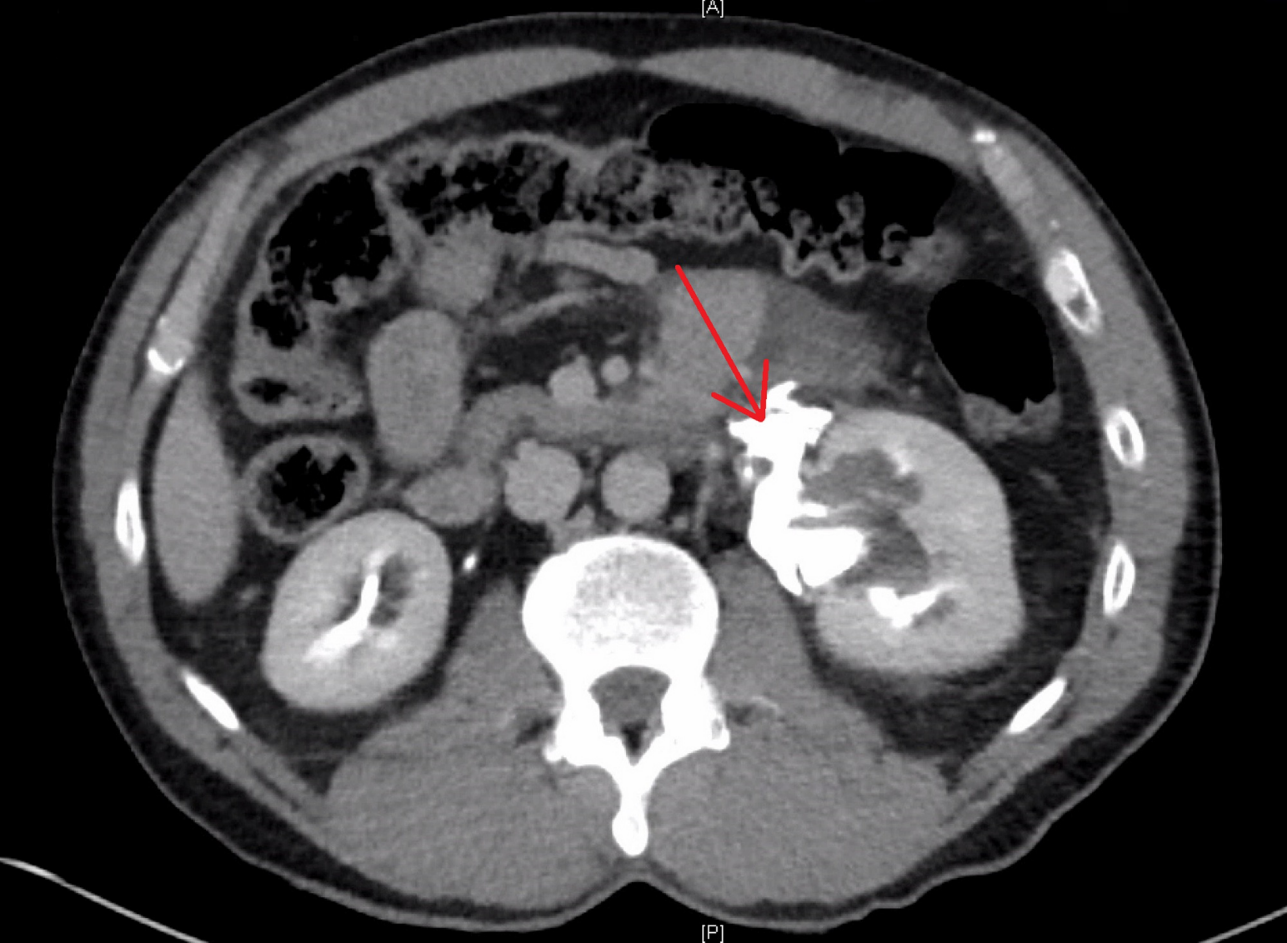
Delayed transverse CT imaging. Area of contrast extravasation denoted by red arrow.
